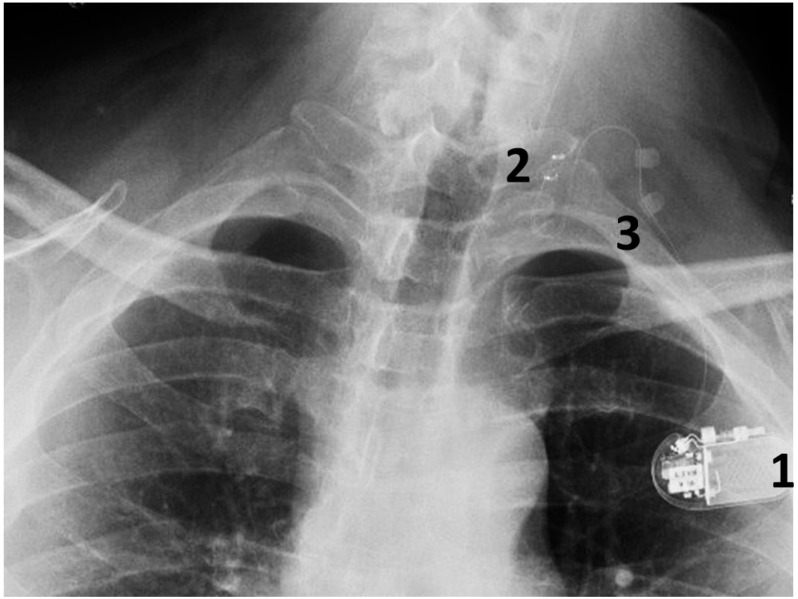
A chest radiograph showing the components of the implanted VNS system: 1. The implantable pulse generator; 2. the electrode contacts on the left vagus nerve; 3. silicone anchors to stabilize the electrode in place.
